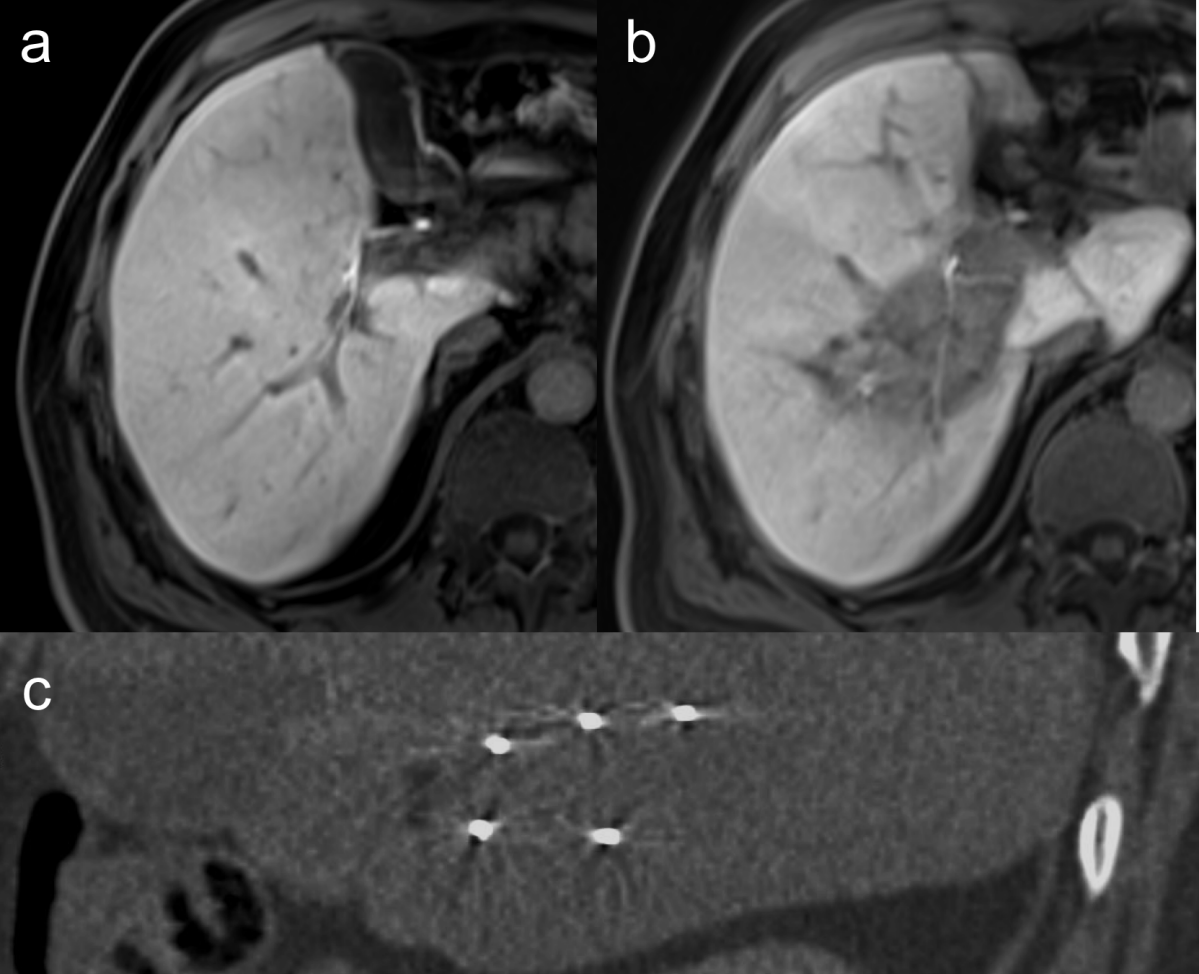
Colorectal liver metastasis in close relationship to the right portal vein. (A) Late phase MRI shows complete ablation (B) after stereotactic placement of 3 IRE electrodes above and 2 below the portal vein (C).
